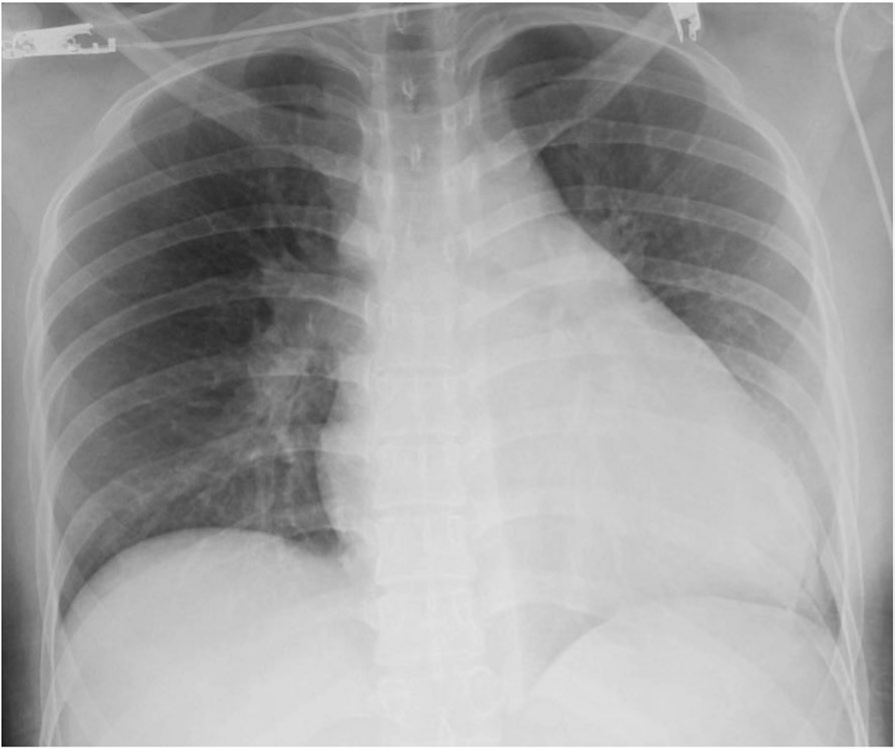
Preoperative chest radiograph showing remarkable enlargement of the cardiac shadow
